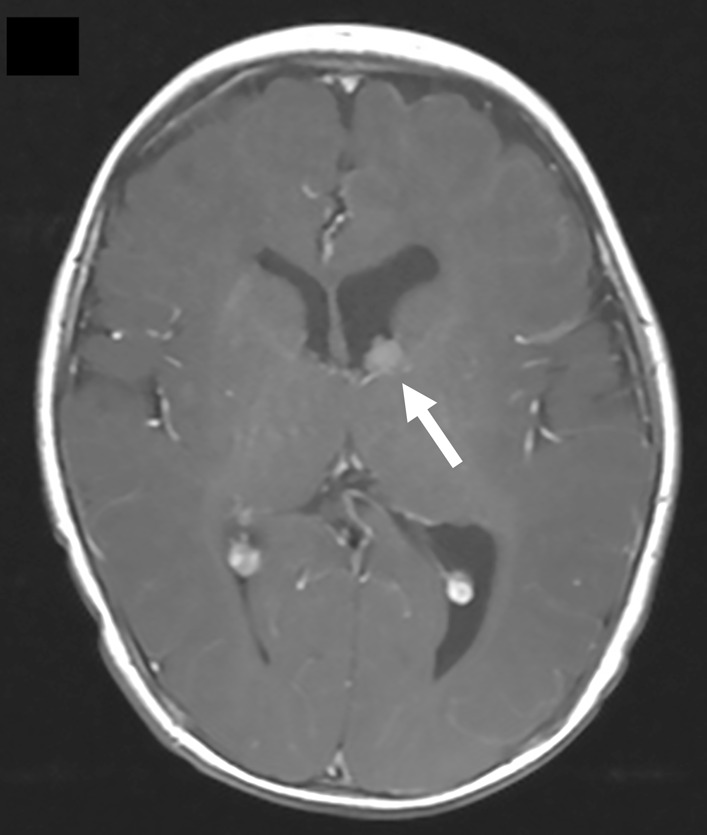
Case 11: Figure 6. Brain MRI T1 SE post gadolinium contrast. Avidly enhancing mass at the anterior left lateral ventricle suspicious of subependymal giant cell astrocytoma (arrow)
